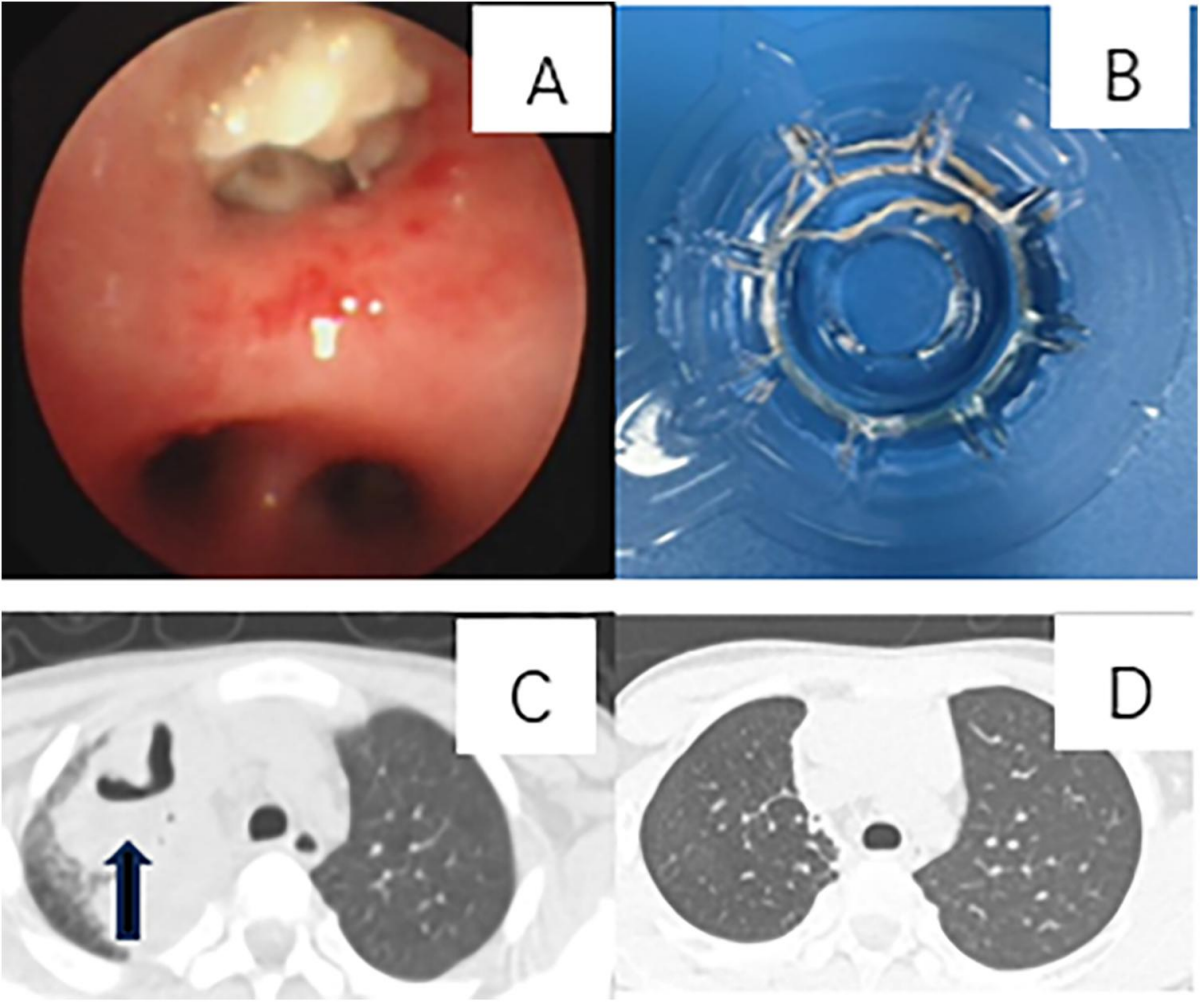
A case of plastic bronchitis associated with Mycoplasma pneumoniae infection presenting with temporary necrotizing pneumonia and subsequent complete recovery. A 10-year-and-3-month-old boy was diagnosed with Mp infection. He underwent bronchoscopy interventions on days 10, 16, and 21 post-onset and was treated with levofloxacin and methylprednisolone at a daily dosage of 10 mg/kg. The patient developed temporary necrotizing pneumonia, followed by complete recovery. (A) Bronchoscopy revealed an airway obstruction in the right upper lobe, characterized by a bronchial cast. (B) A bronchial cast observed during bronchoscopy. (C) A CT scan on day 40 of the illness course showed necrotizing pneumonia in the right upper lobe, with obstruction of the bronchi in the same region. (D) A follow-up CT scan, conducted 7 months after onset, demonstrated complete recovery.
